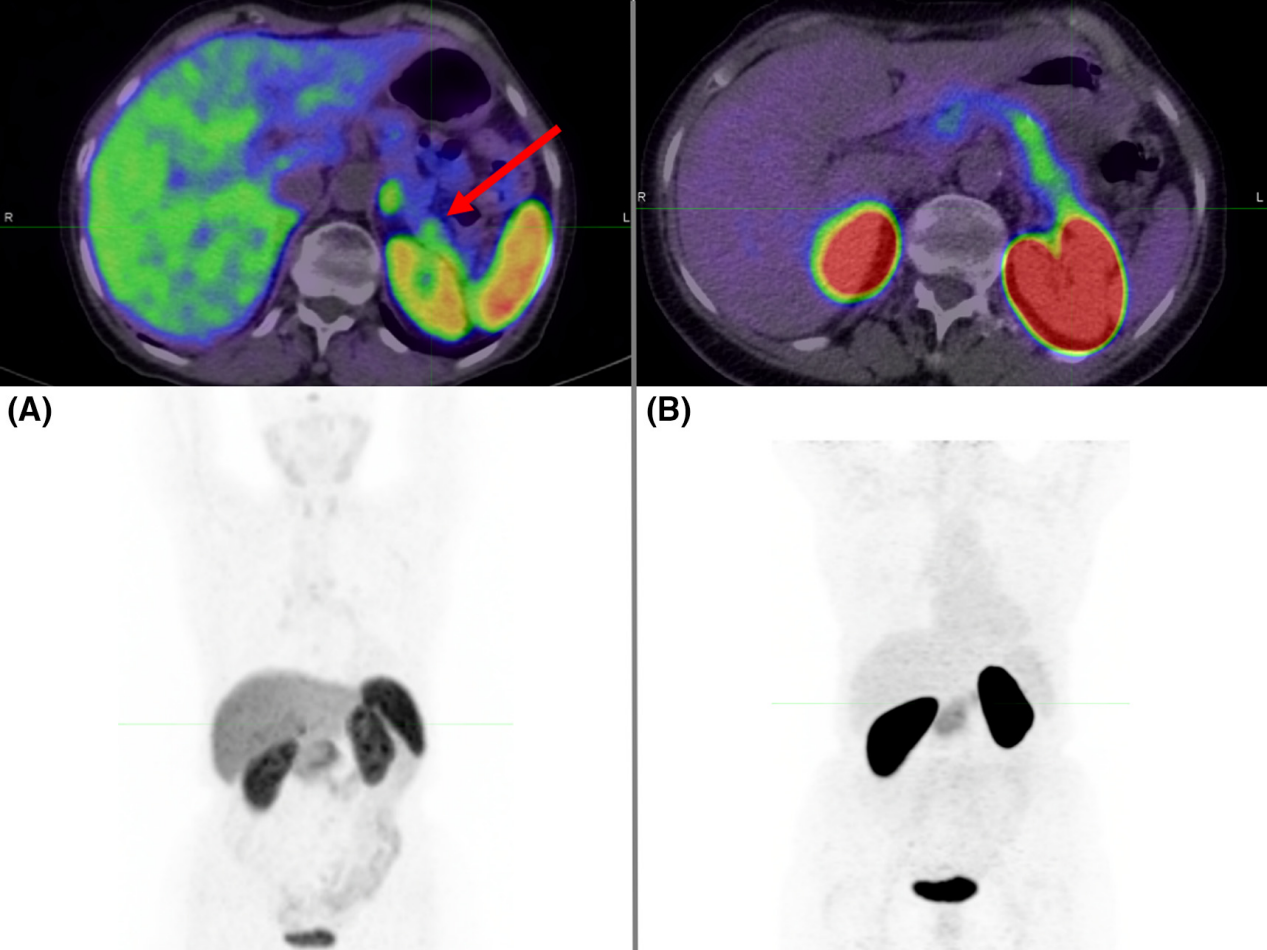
(A) ^68^Ga‐DOTATATE PET/CT. Mild focal uptake in pancreatic tail suspicious for insulinoma but difficult to delineate from adjacent renal activity. (B) ^68^Ga‐DOTA‐exendin‐4 PET/CT demonstrates diffuse pancreatic uptake higher than what would be expected physiologically, suggestive of nesidioblastosis without focal intense uptake to indicate insulinoma. Again, intense renal uptake potentially compromises assessment of the pancreatic tail.
